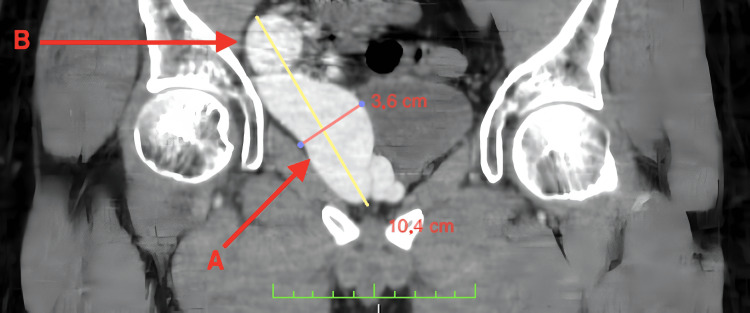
CT angiography. A coronal section of the pelvis. Note the craniocaudal extension of the venous aneurysm (A: confluent venous branch, B: internal iliac vein).
